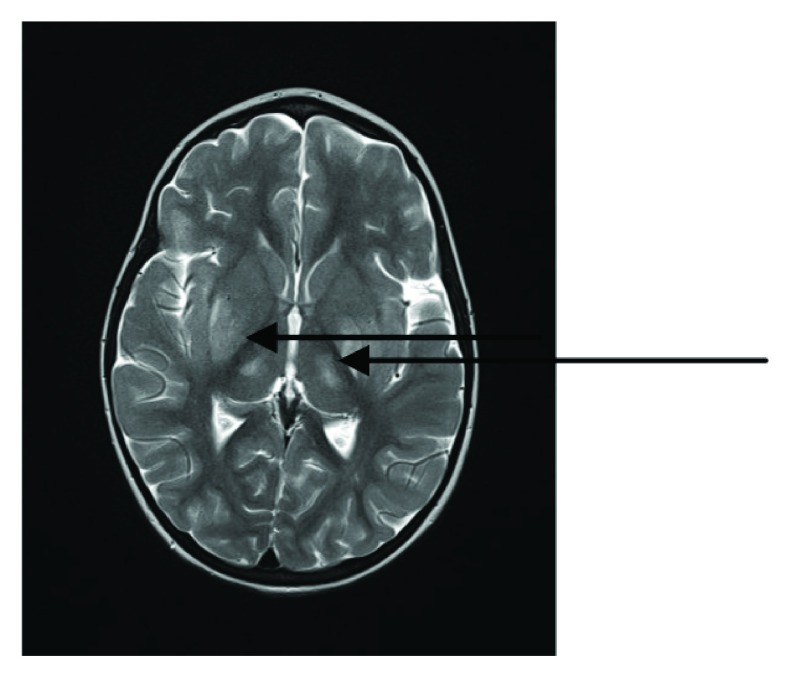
MRI scan of brain recorded on the 12th day of disease: bilateral hyperintensity in lateral thalamic regions (arrows).
